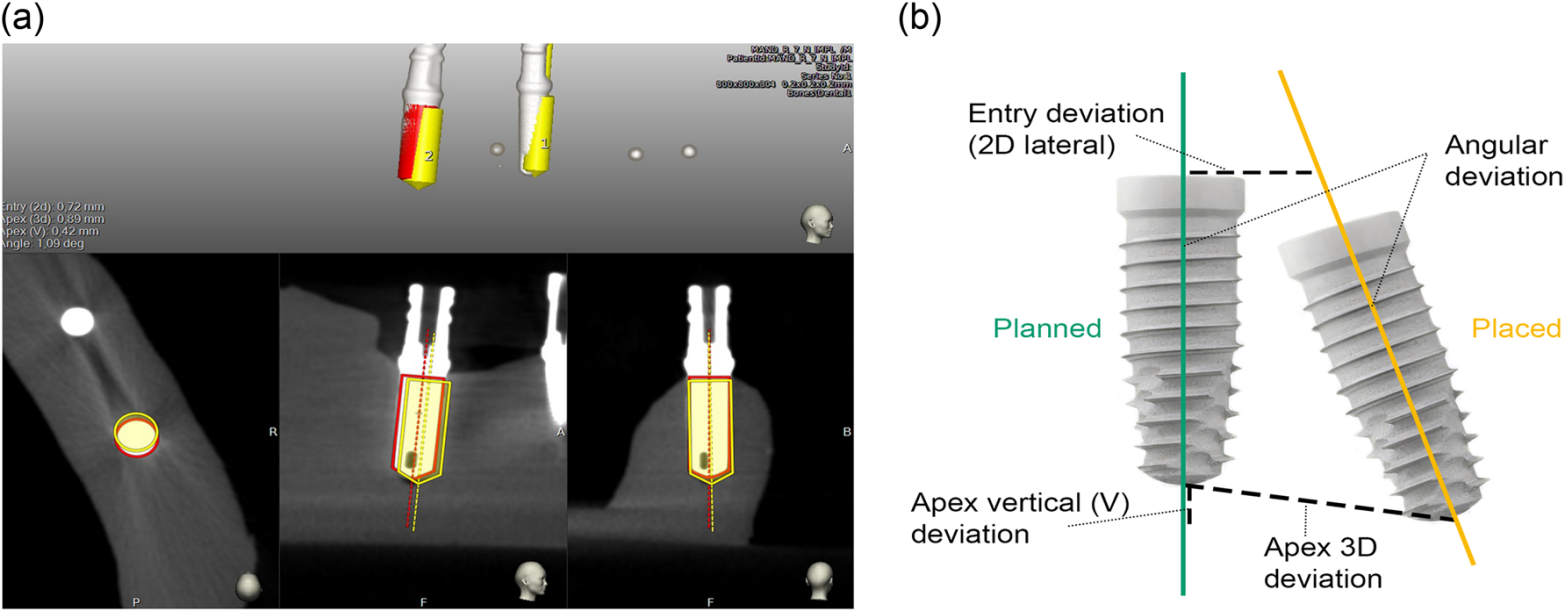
(a) Software (EvaluNav) superimposition of pre‐ and postoperative CBCT; (b) measured deviations.
